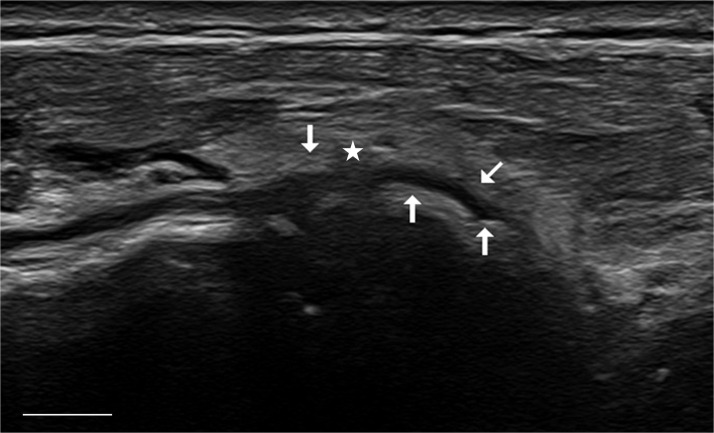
High-resolution ultrasound of posterior interosseus nerve in the distal aspect of the supinator tunnel. The posterior interosseous nerve is depicted in longitudinal view (small arrows) from proximal (left) to distal (right). Note the main compression site of the nerve (star) due to an exostosis of the radius with slight nerve enlargement located proximally. Scale bar: 5 mm.
